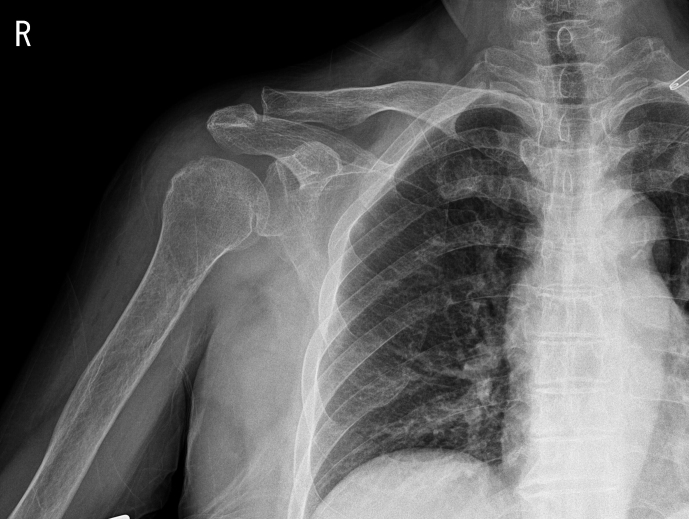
Plain radiograph showing osteoarthritic changes in the right acromioclavicular joint and soft-tissue swelling around the right shoulder.
